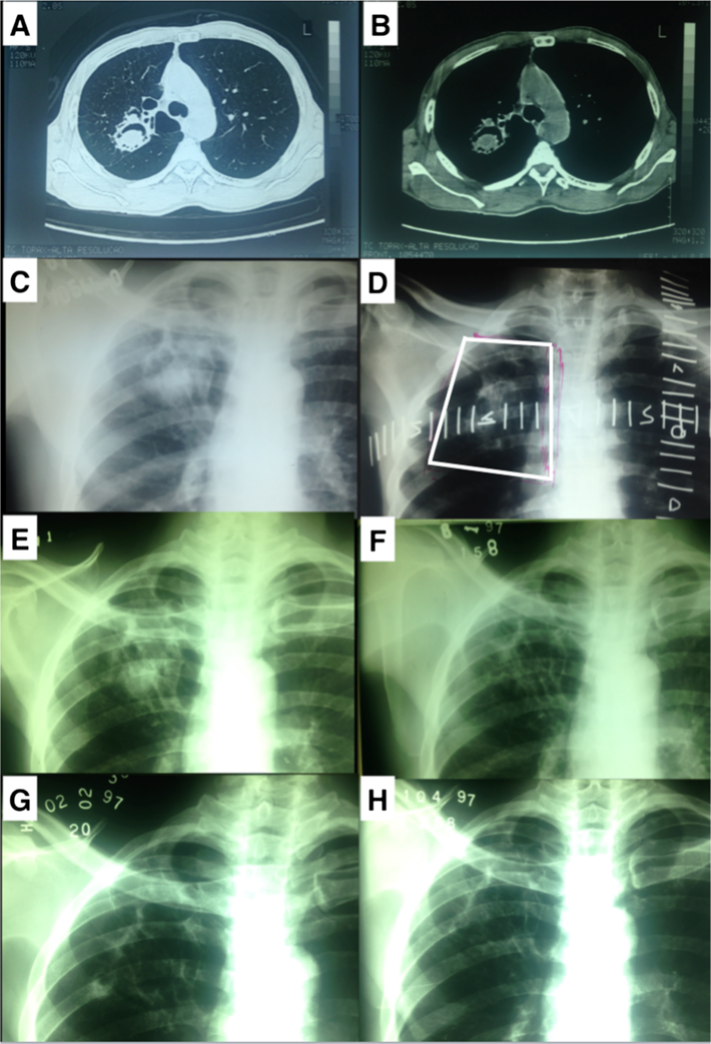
a 46-year-old man with a diagnosis of right superior lobe aspergilloma treated in August 1997. a) and b) Diagnostic computed tomography. c) Diagnostic x-ray. d) Localization x-ray. e) One-month follow-up x-ray. f) Five-month follow-up x-ray. g) Six-month follow-up x-ray. h) Eight-month follow-up x-ray
